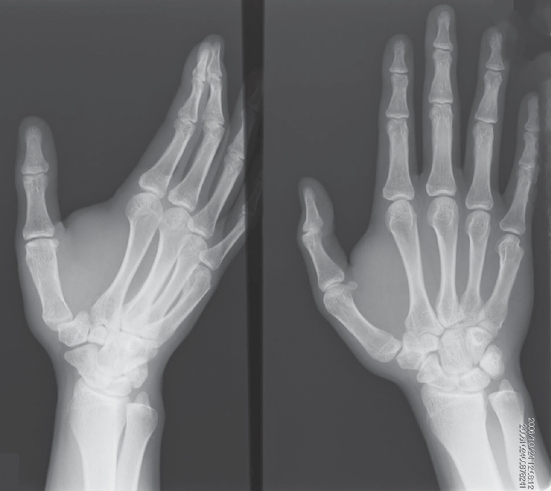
Thallium-201 scintigraphy shows high uptake in the early phase and no wash-out on the delayed phase.
